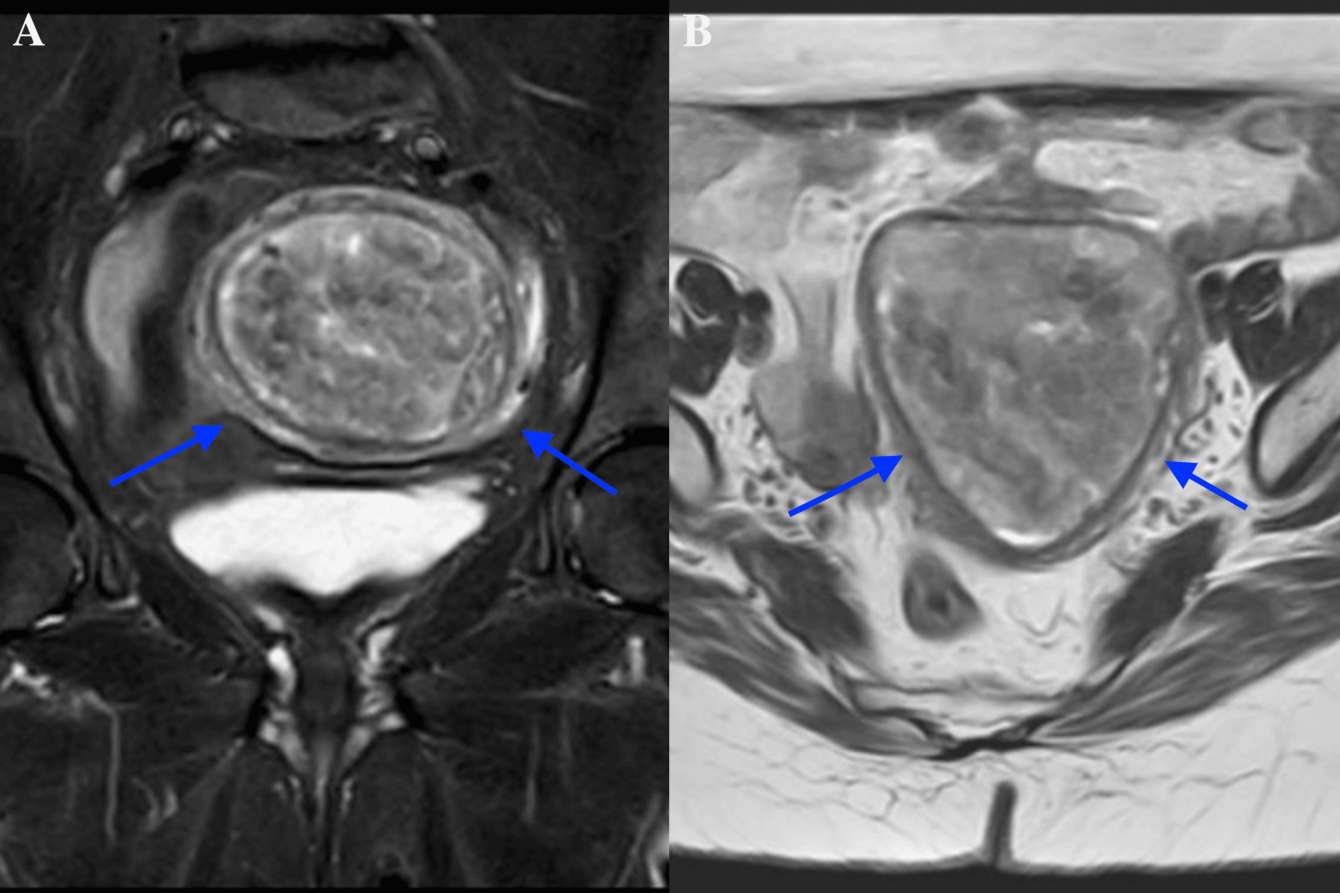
Pelvic MRI showing (A) a coronal view of a large, well-defined endometrial mass with irregular borders and (B) an axial view emphasizing its infiltrative nature, indicative of a malignant process
